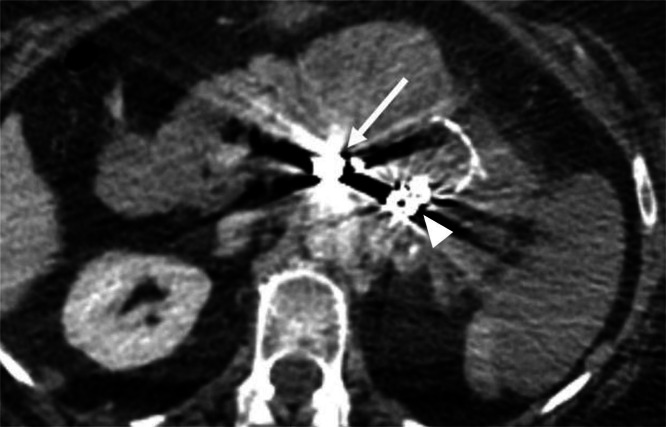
Follow-up contrast-enhanced CT confirming complete exclusion of the pseudoaneurysm (arrow indicates the deployed coils). Coils migrated into the pseudoaneurysmal sac are also visualized (arrowhead).
